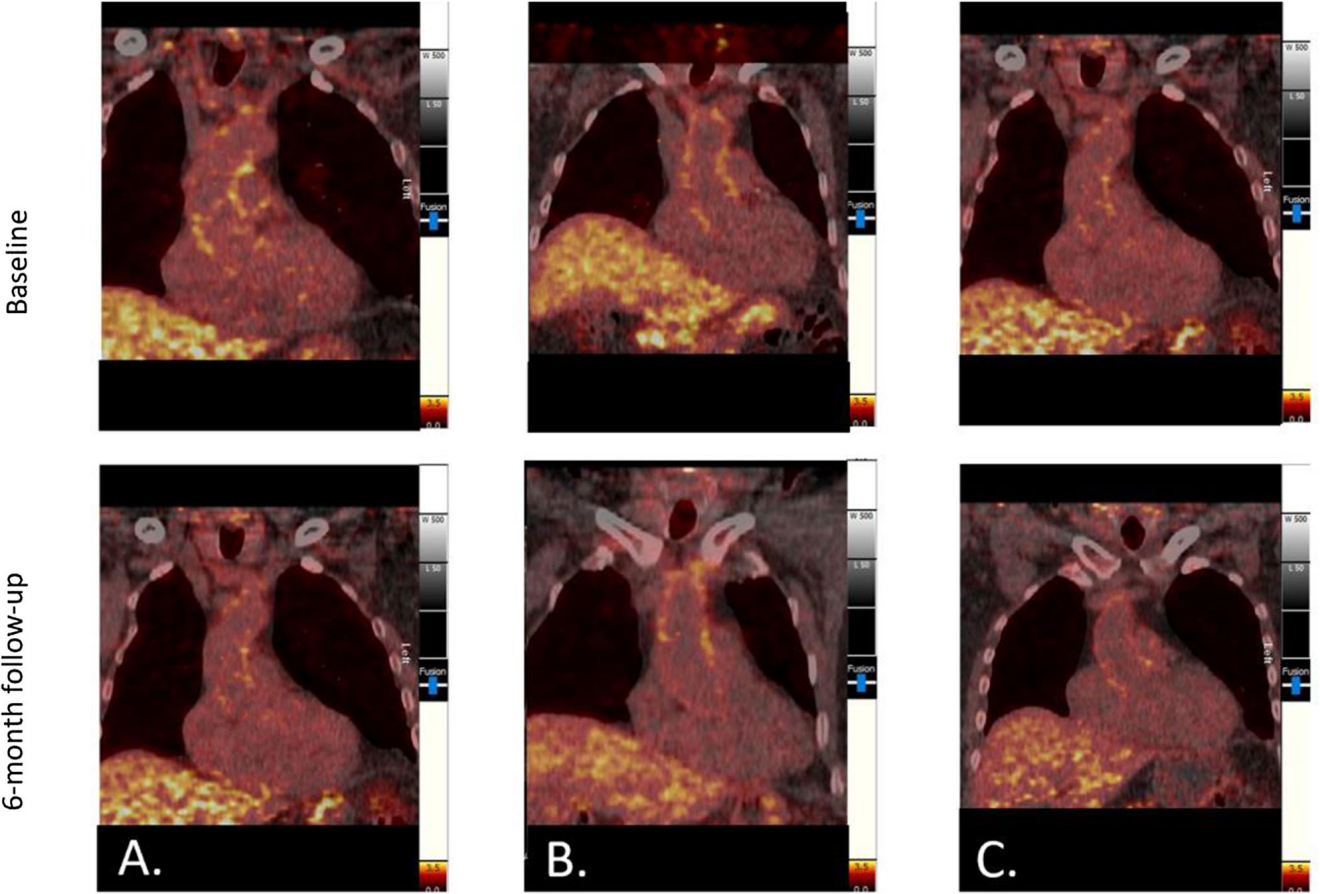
FDG images showing change in ascending aorta FDG uptake from baseline to 6-month follow-up in (A) patients with PsA and/or PsO on biologic agents, (B) patients with PsO on non-biologic agents, and (C) control patients with non-inflammatory arthritis. *FDG*, F-18-fluorodeoxyglucose; *PsA*, psoriatic arthritis; *PsO*, psoriatic disease
